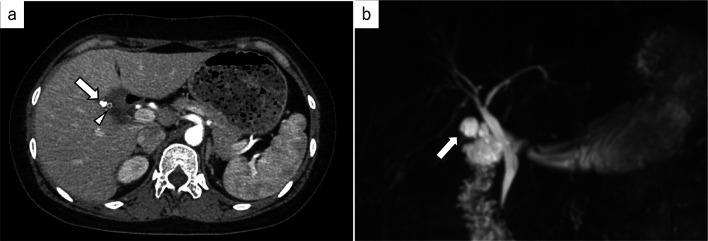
Abdominal contrast-enhanced CT and MRCP images. a Contrast-enhanced axial CT image: a calculus is seen in the remnant cystic duct (arrowhead). The staple line of the prior subtotal-cholecystectomy (arrow) is apparent on the fundal side of the remnant gallbladder. b MRCP image: no choledocholithiasis or intrahepatic bile duct dilation is observed. The arrow indicates the remnant gallbladder
